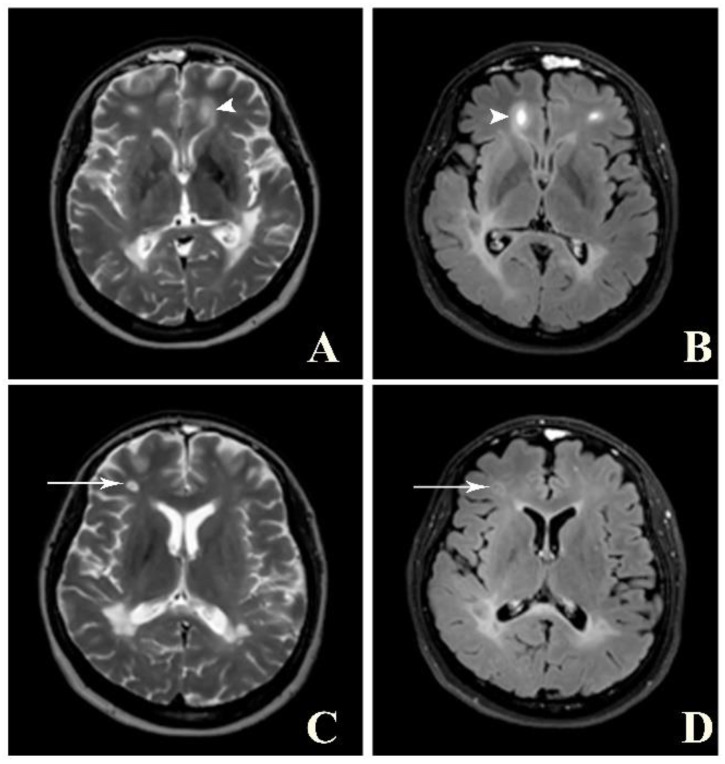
Balo’s concentric sclerosis. A and B: Axial T2W (A) and post-contrast FLAIR (B) images show a few lesions with alternating band of high and low signal intensity at bilateral frontal lobes (arrowhead). C and D: Axial T2W (C) and post-contrast FLAIR (D) images of the same patient show faint central and peripheral enhancement at the right frontal lobe lesion (arrow).
